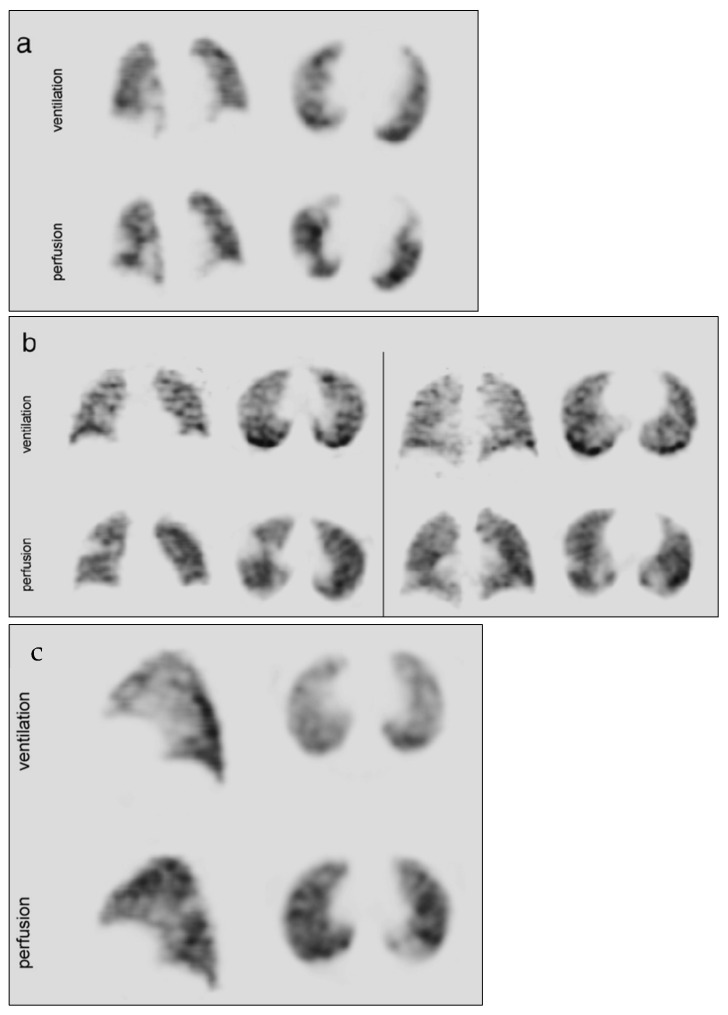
(a) A 63-year-old male with a 10-h history of right-sided pleuritic chest pain. V/Q SPECT showed multiple asymmetric wedge-shaped perfusion defects in reference to PE. (b) A 37-year-old male who presented with a swollen leg and chest pain. SPECT showed multiple asymmetric wedge-shaped perfusion defects in reference to PE. (c) A 31-year-old female who presented with shortness of breath, pleuritic chest pain, and two episodes of haemoptysis. V/Q SPECT showed a single subsegmental asymmetric perfusion defect in the left lung. Computed tomography pulmonary angiography performed the next day showed no PE, but lingular atelectasis, pericardial thickening, and a small pericardial effusion. Reprinted/adapted with permission from Ref. [35]. 2014, Elsevier.
